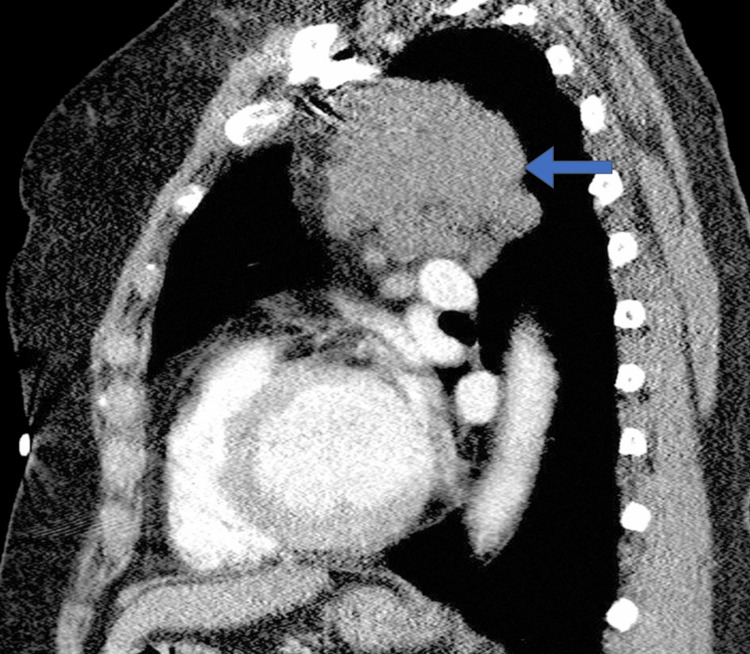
Sagittal CT image demonstrates a lobulated cystic lesion (arrow) in the superior mediastinum
